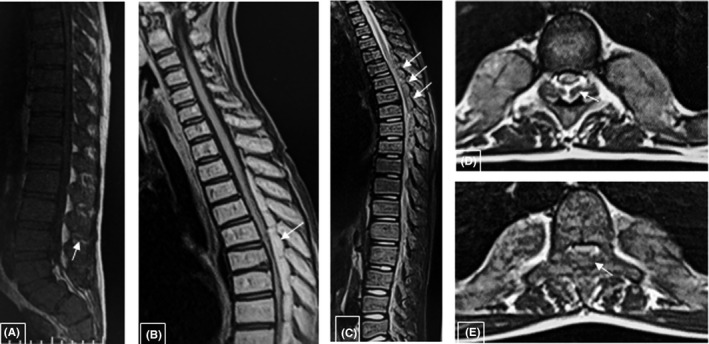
MRI sagittal T1 WI (A and B), sagittal T2WI ^©^, axial T2 WI (D and E) shows diffusely hypointense marrow (A), paraspinal and epidural masses extending from T2 to L2 causing cord compression regarding T5 and T6 (arrows), and an anterior epidural mass regarding S1 causing the compression of the filum terminale (2A arrow)
